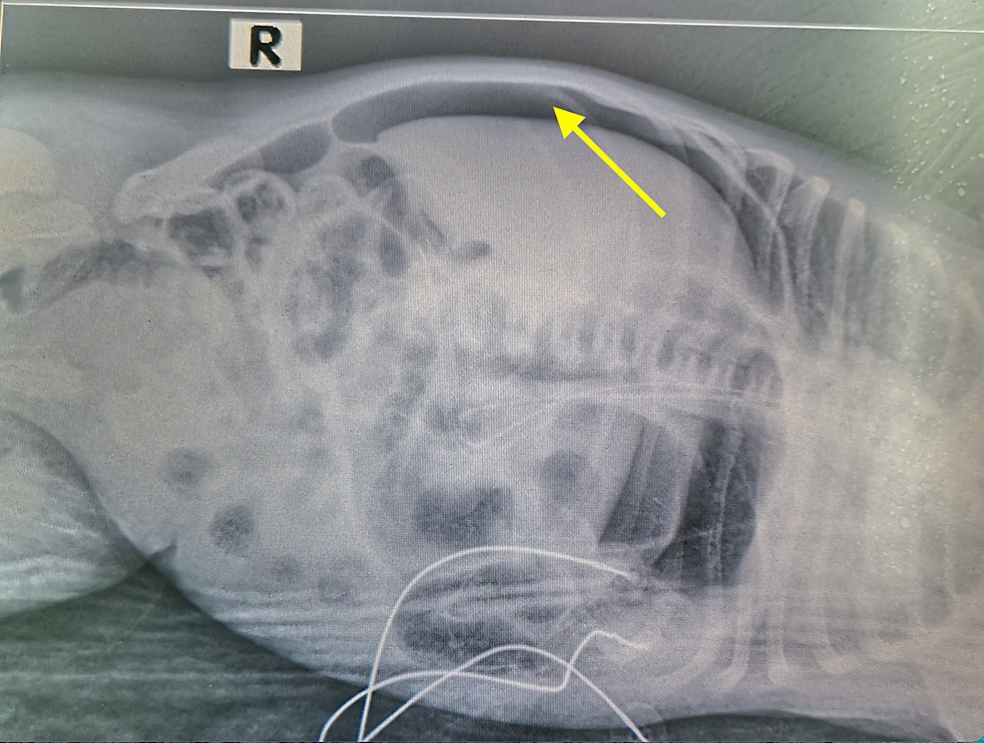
A lateral cross-table radiograph showing free gas above the liver (yellow arrow), which is suggestive of intestinal perforation.
